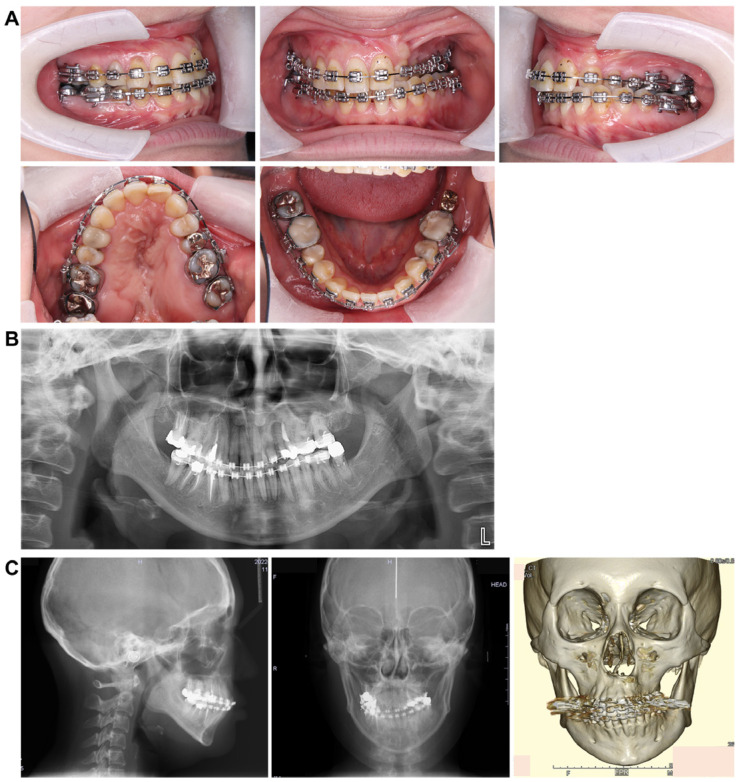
Preoperative intraoral photograph and radiographs and CT. (A) Intraoral photographs. (B) Panoramic radiograph. (C) Cephalograms and CT.
